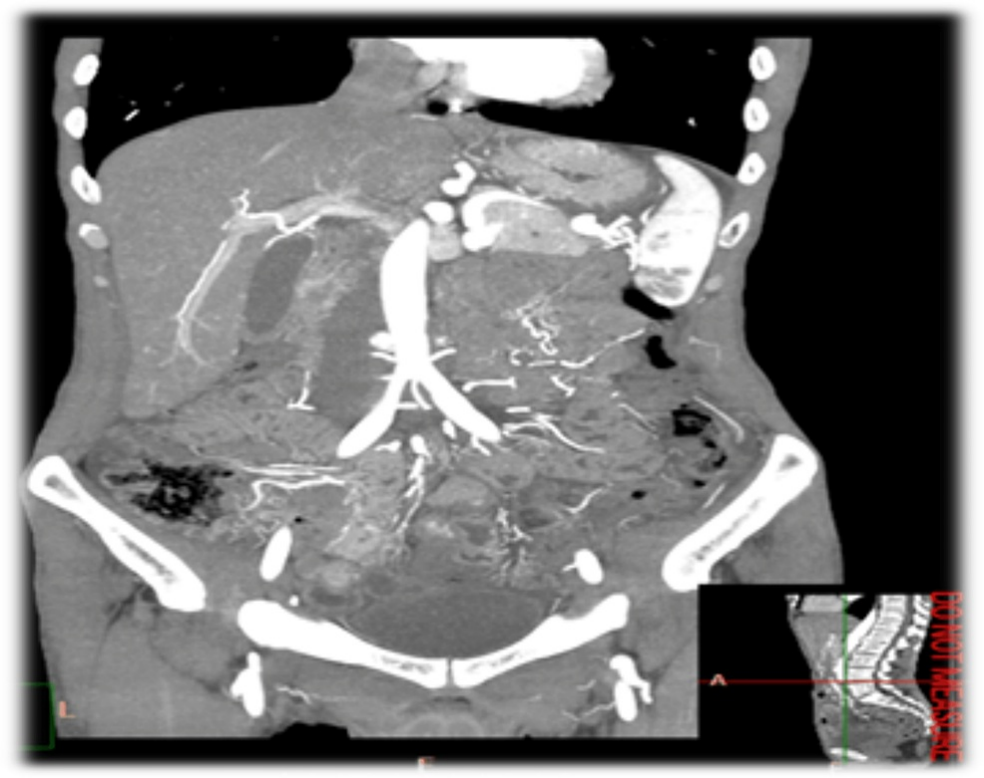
Abdominal CT angiogram Imaging showed patent abdominal vessels, normal angulation, and no signs of mesenteric artery obstruction
